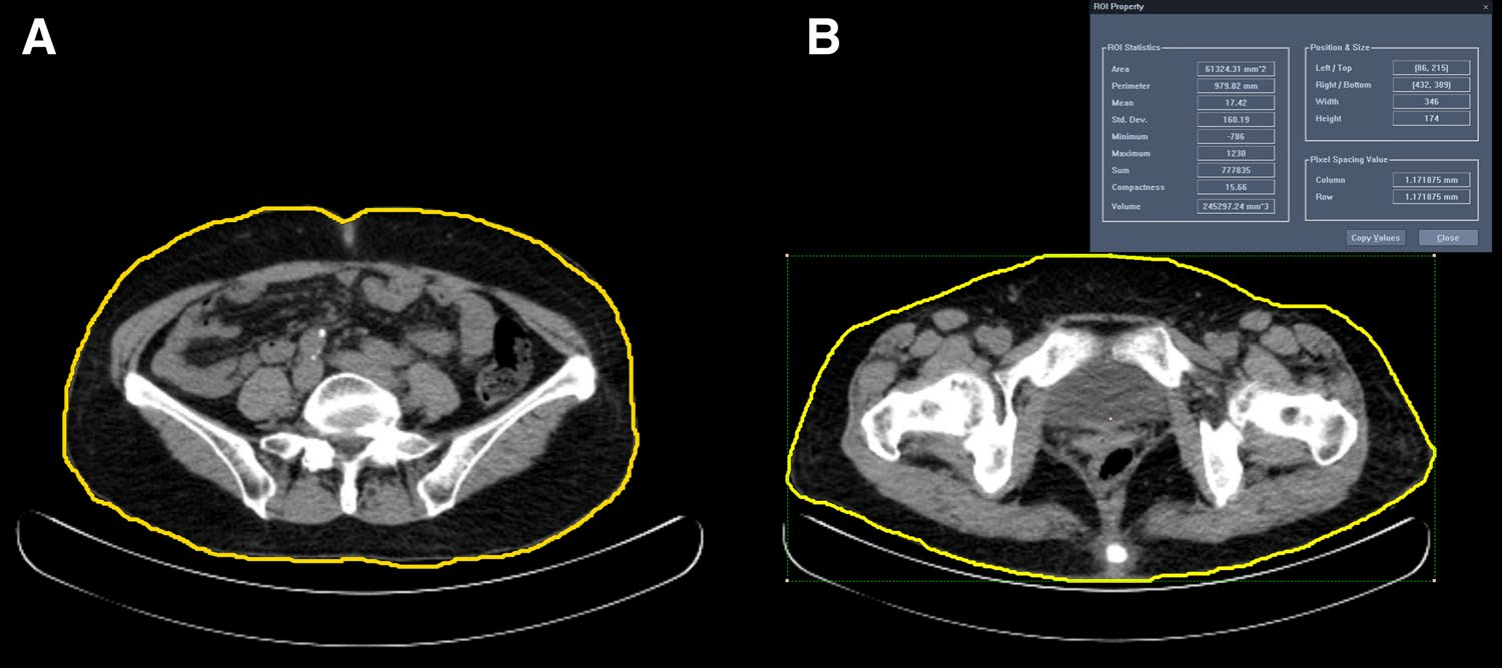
An example of region of interest (ROI)s on computed tomography. ROIs are used to obtain the waist circumference (WC) and hip circumference, and are manually drawn on the transaxial non-enhanced computed tomography image at the umbilicus level for WC (A) and the greater trochanter level of the femur (B).
